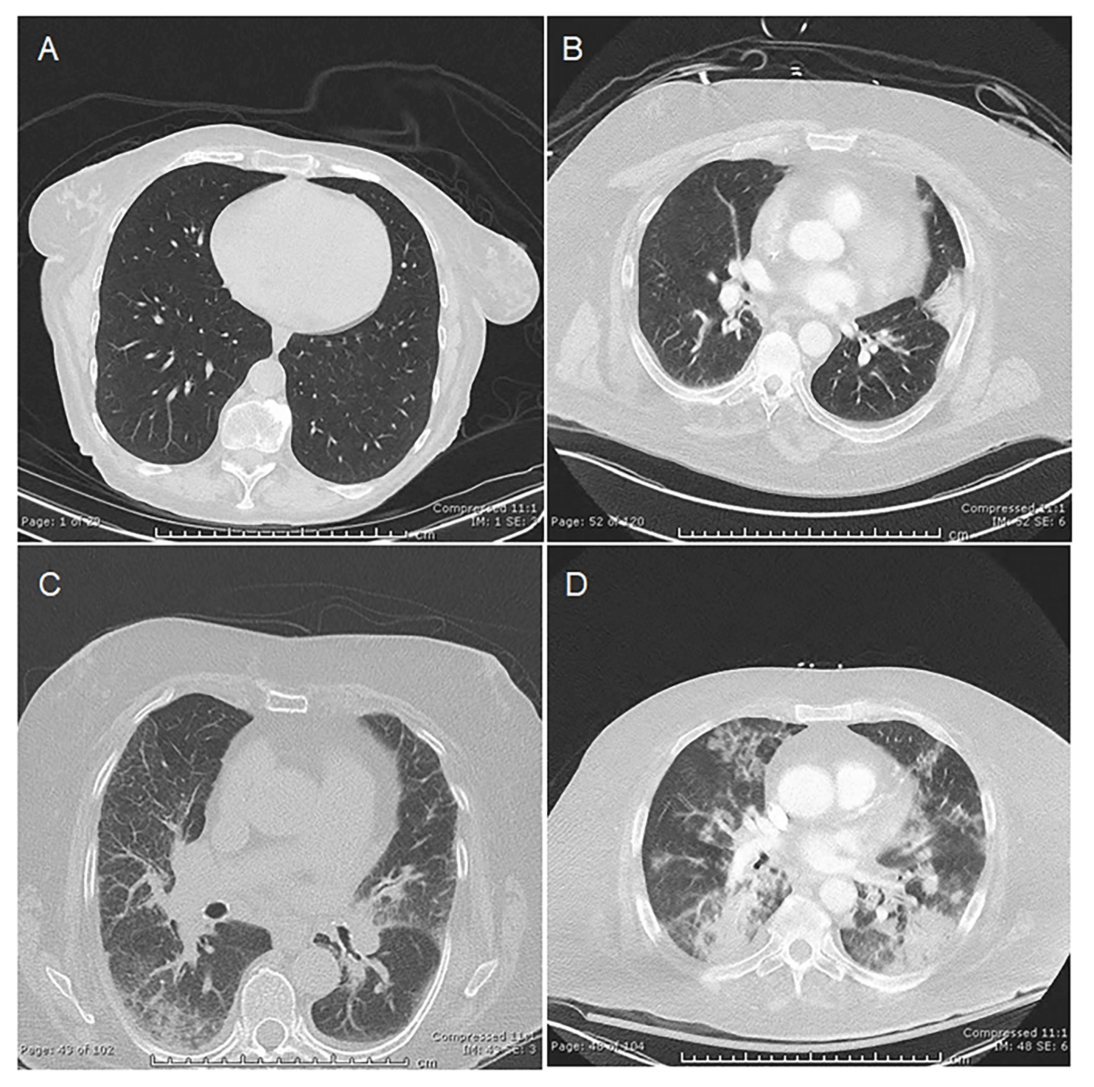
Representative chest CT scans in influenza patients. A) Normal, B) Mildly abnormal, C) Moderately abnormal, and D) Severely abnormal
